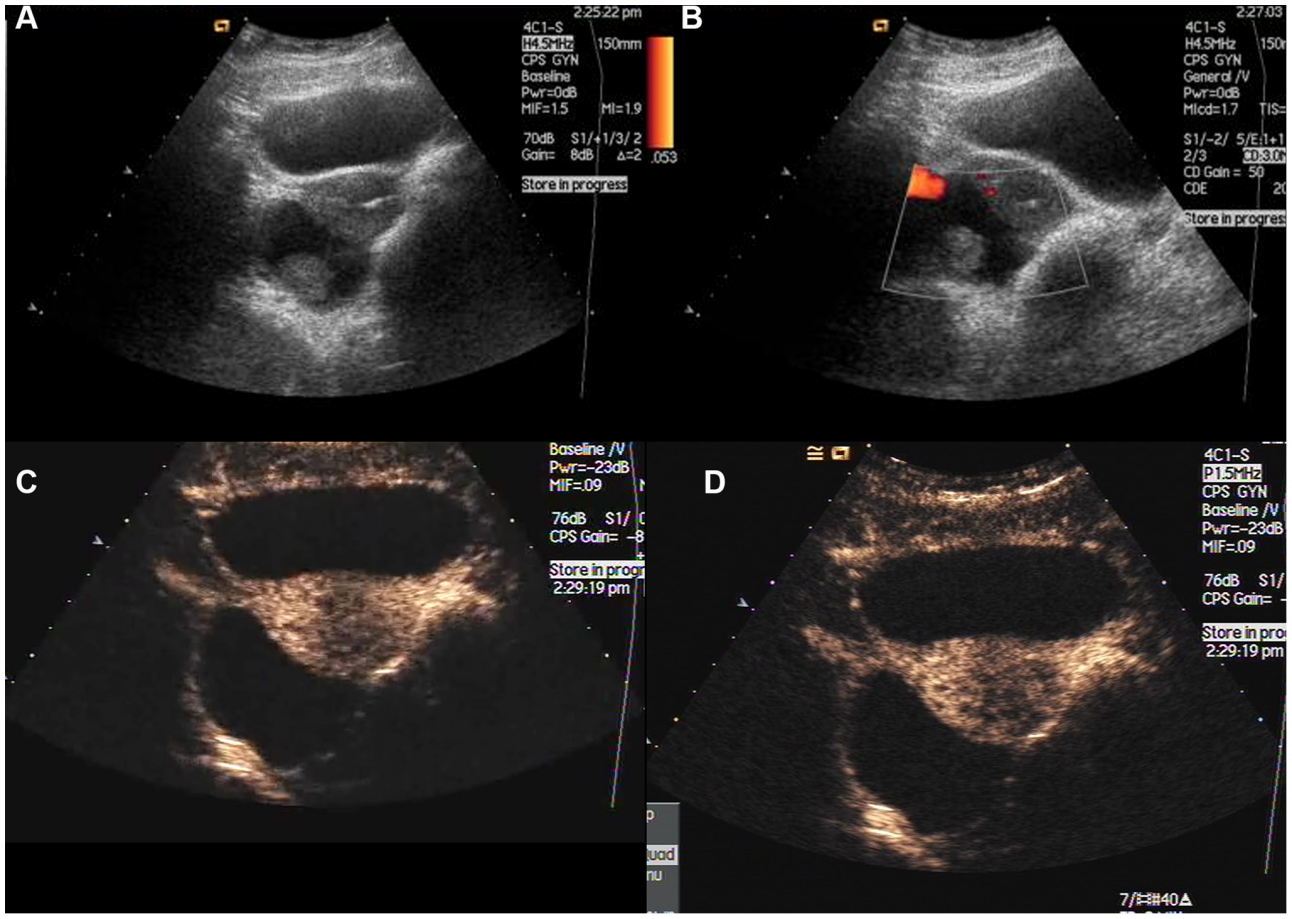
A 21-year-old woman with endometrial cyst. A: Gray-scale sonogram shows a 6.2-cm inhomogeneous mass with solid projection at the right of uterus. B: Power Doppler shows minimal vascularization. C: CEUS scan at 9 s shows later enhancement in comparison with myometrium (arrow), no agent perfusion inside. D: CEUS scan at 17 s shows homogeneous ringed (arrow).
